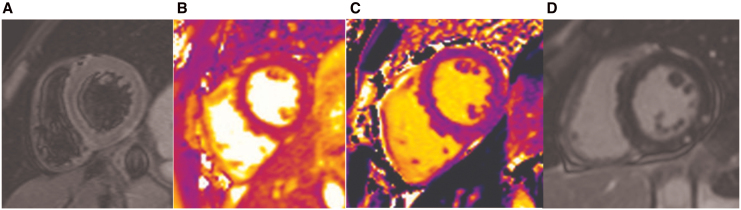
Baseline cardiac magnetic resonance imaging. The combination of diffuse native T1 elevation, borderline T2 prolongation, and non-ischaemic subepicardial late gadolinium enhancement supports the diagnosis of acute inflammatory myocarditis. *(A*) T2-weighted STIR sequence. Mid-ventricular short-axis view demonstrating increased subepicardial signal intensity in the inferolateral wall, consistent with myocardial oedema. *(B*) T2 mapping (T2-prepared bSSFP). Mid-ventricular short-axis view showing globally increased T2 values at the upper limit of normal (51 ± 4 ms), suggesting myocardial oedema (normal reference range for males ≈ 45 ± 2 ms). *(C*) Native T1 mapping (MOLLI sequence). Mid-ventricular short-axis view demonstrating diffusely elevated native T1 values (global T1 1107 ± 56 ms), consistent with diffuse myocardial injury and inflammatory involvement (normal MOLLI T1 reference for males ≈ 983 ± 50 ms). *(D*) Late gadolinium enhancement (PSIR gradient-echo sequence). Mid-ventricular short-axis view demonstrating focal non-ischaemic enhancement with a subepicardial distribution in the basal inferior and inferolateral segments of the left ventricle.
